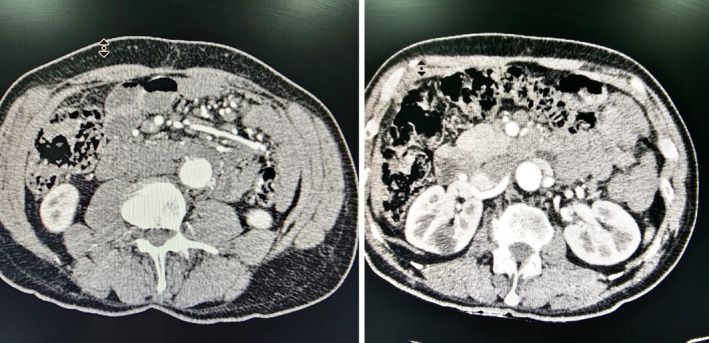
Computed tomography angiography scan demonstrating no complications 1 year after operation, with a patent in‐situ graft.
